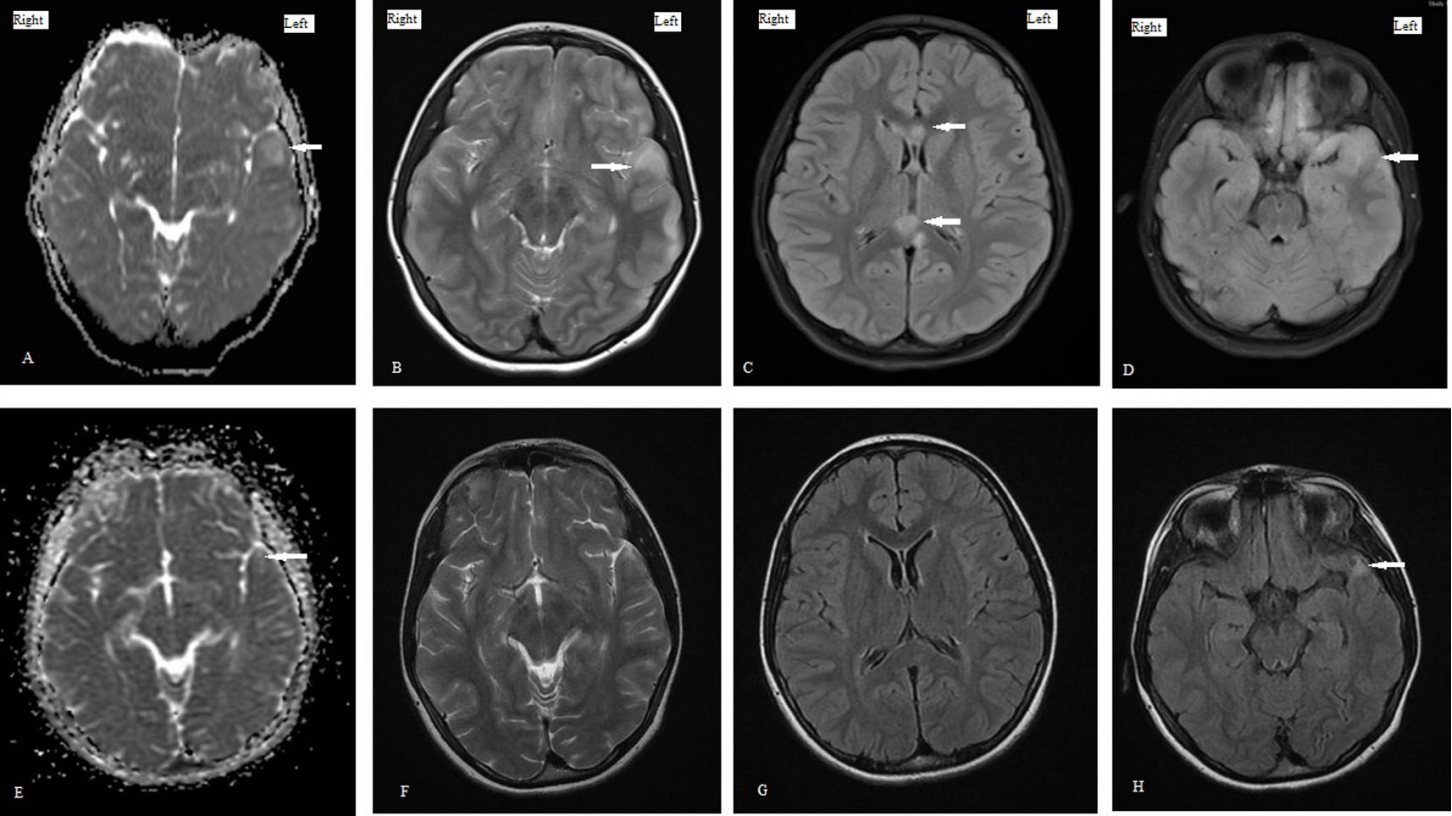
A-D: Initial MRI brain images showing changes of diffuse axonal brain injury; A - ADC sequence showing edema, B - T2 weighted images showing hyperintense left temporal lobe, C - FLAIR sequence showing hyperintense signals in corpus callosum, D - FLAIR sequence showing hyperintense left temporal lobe suggestive of edema. E-H: Follow up MRI brain images; E - ADC sequence showing residual minimal bright signal in left temporal area, F - T2 weighted sequence showing significant resolution of left temporal edema, G - FLAIR image showing resolution of changes in corpus callosum, H - FLAIR sequence showing residual hyperintense lesion in left temporal lobe.
